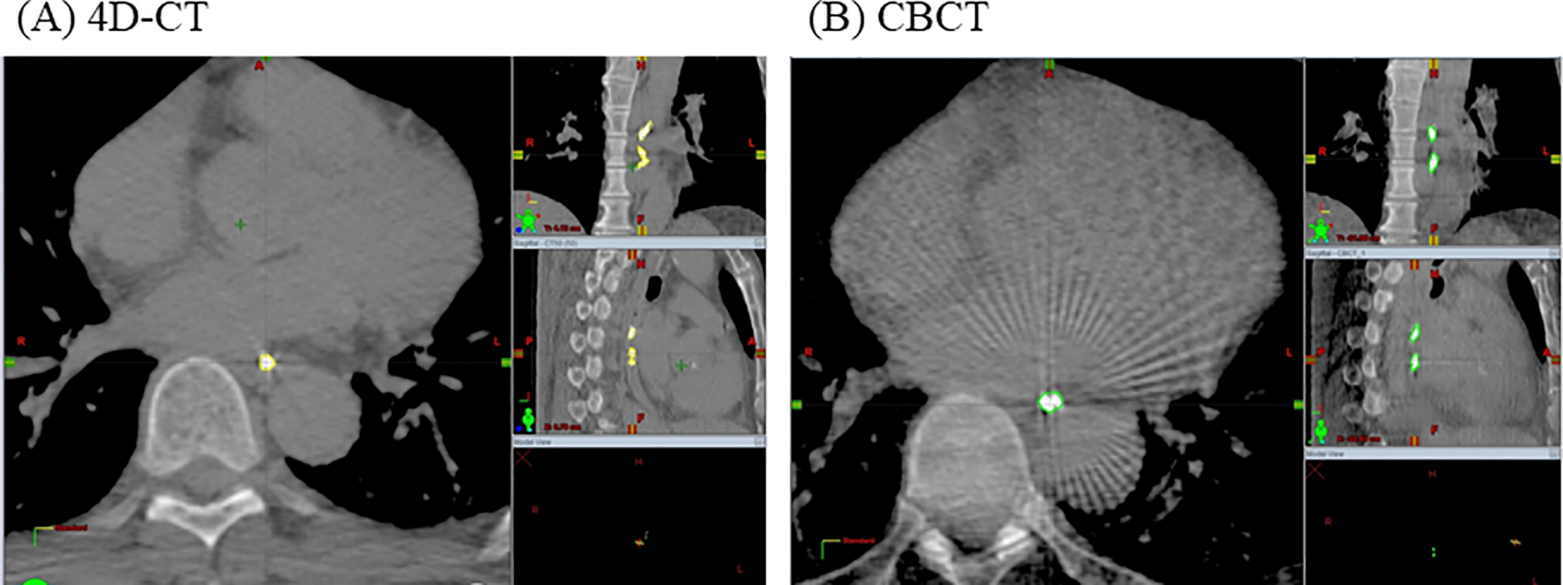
Contouring of metallic markers. Metal markers, placed by endoscopy to identify tumor location, were contoured in (A) four-dimensional computed tomography (4D-CT) and (B) cone beam CT (CBCT).
